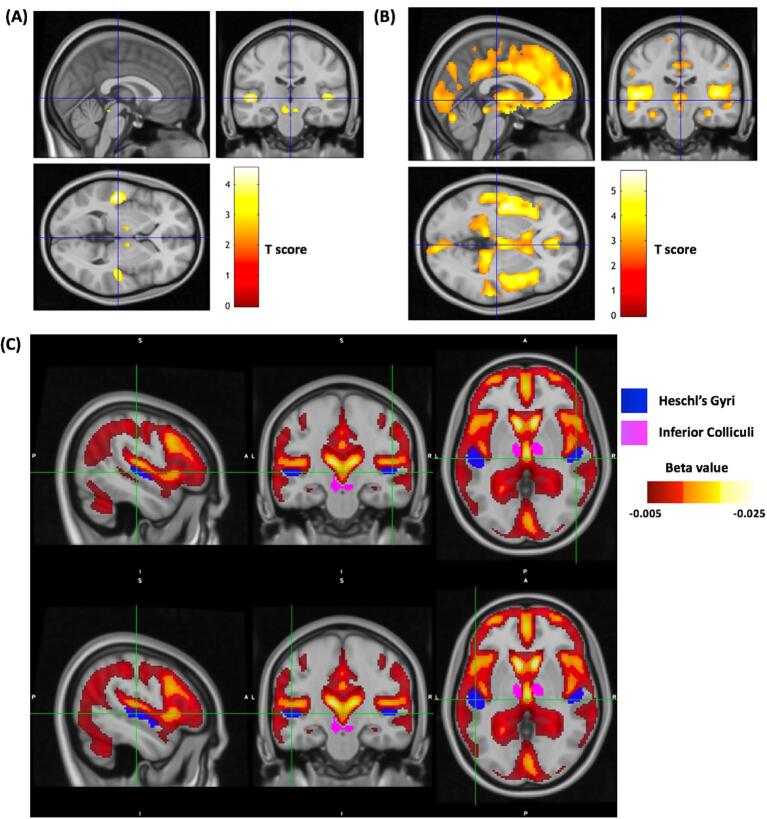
Effects of hearing loss on glucose metabolism. Glucose hypometabolism patterns in bilateral Heschl’s Gyrus and midbrain auditory pathway (e.g., inferior colliculus) are apparent in (A) with age correction and (B) without age correction at a cluster-forming height threshold p-value = 0.005 (uncorrected) with sagittal, axial and coronal sections at x  = 0, y = 22, z = 1. Colour scale gives Student's *t* statistic for the comparison between HL and non-HL. Years of education and sex are included as covariates in SPM two-sample *t*-test for both. (C) shows comparison of effect of aging and the regions of interest selected for GWAS for right hemisphere (top) and left hemisphere (bottom). Regions of interest include bilateral Heschl’s gyrus in blue and inferior colliculus in magenta with sagittal, axial and coronal sections at x = ±50, y = ±20, z = 2. Colour scale gives beta coefficient (*β_c_*) for age in linear regression model for glucose uptake SUVR in all subjects. (For interpretation of the references to colour in this figure legend, the reader is referred to the web version of this article.)
